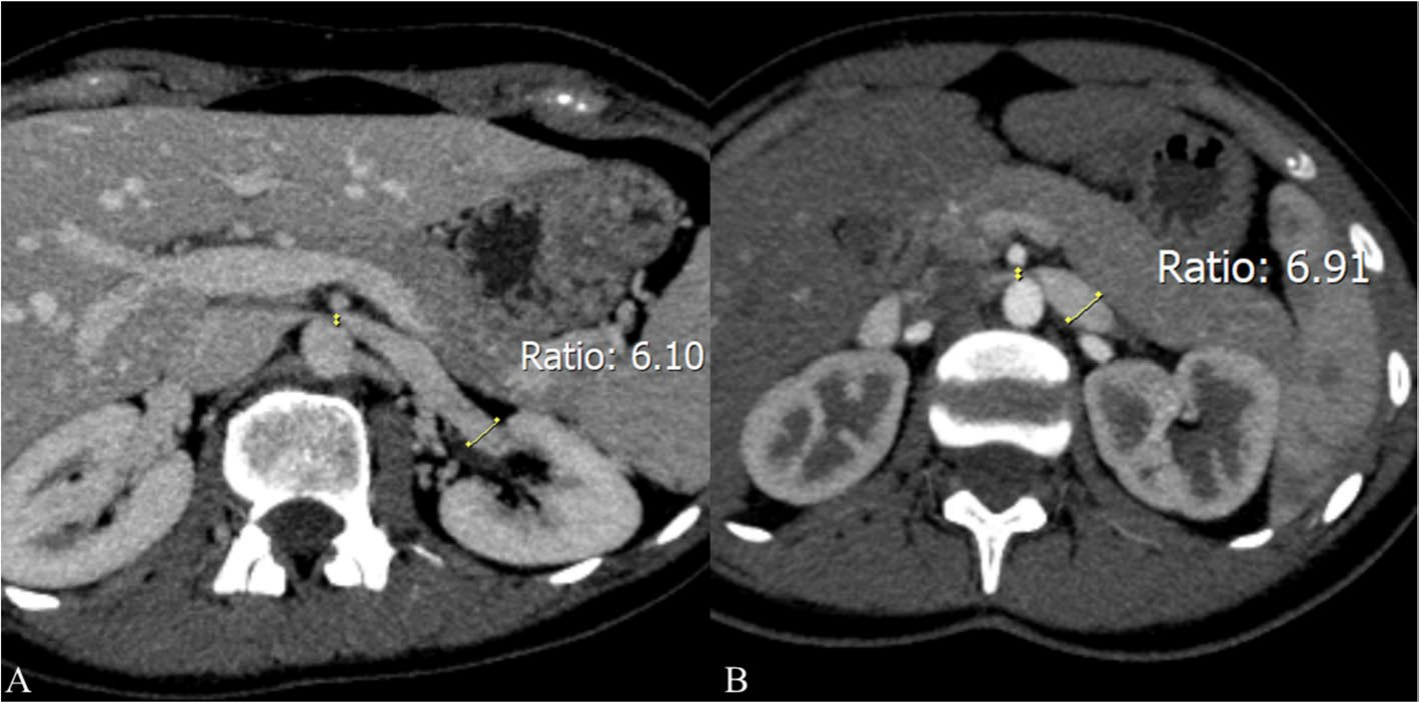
CT axial planes of the same patients with the ratio of the LRV diameter at the hilar and aorto-mesenteric level (the ratio > 5 indicates the presence of NCS): A portal venous phase – the ratio is 6.10; B arterial phase – the ratio is 6.91
